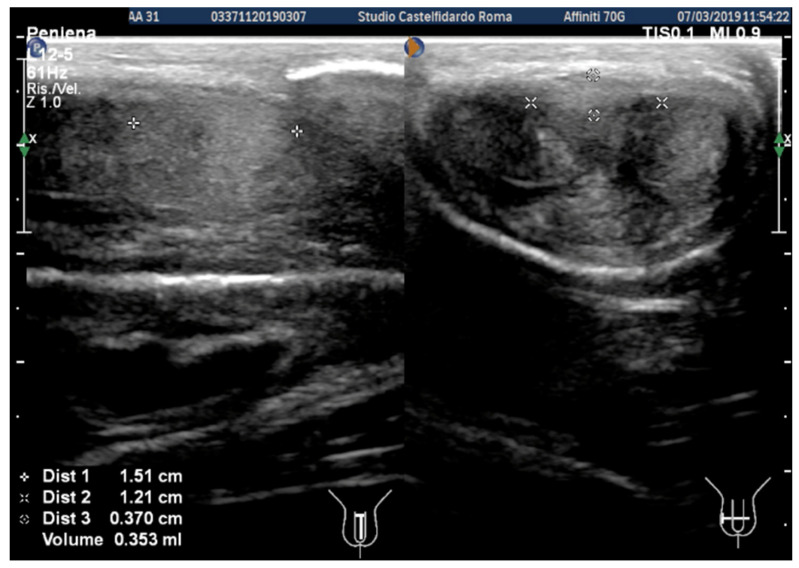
Ultrasonography of the penis before treatment (longitudinal and transverse views).
